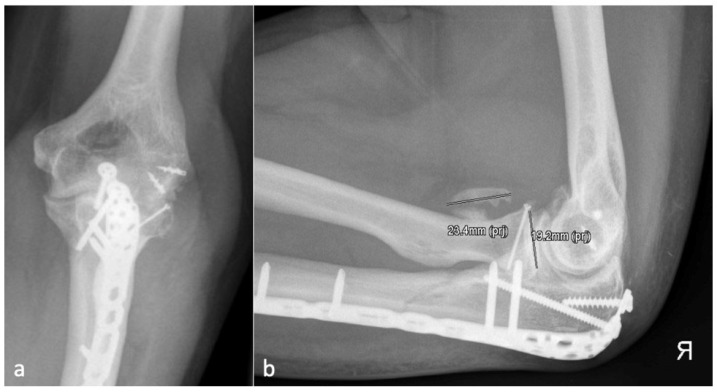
(a) shows the AP and (b) shows the lateral X-ray with an anterior HO (23.4 mm) which is larger than the diameter of the radial head (19.2 mm) and represents an HO 2a.
